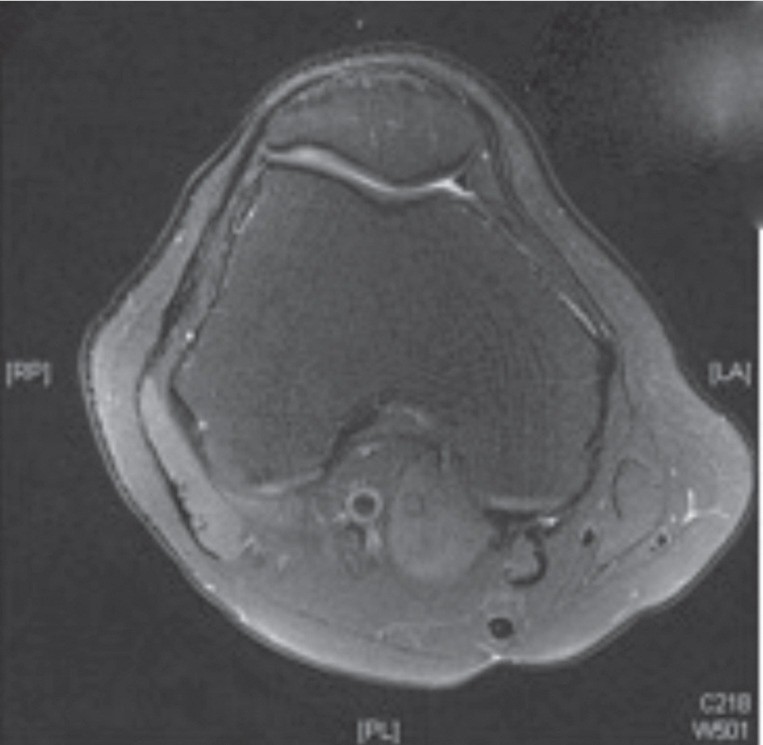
Axial cut of the MRI did not reveal the cyst or other abnormal signal over the iliotibial band.
